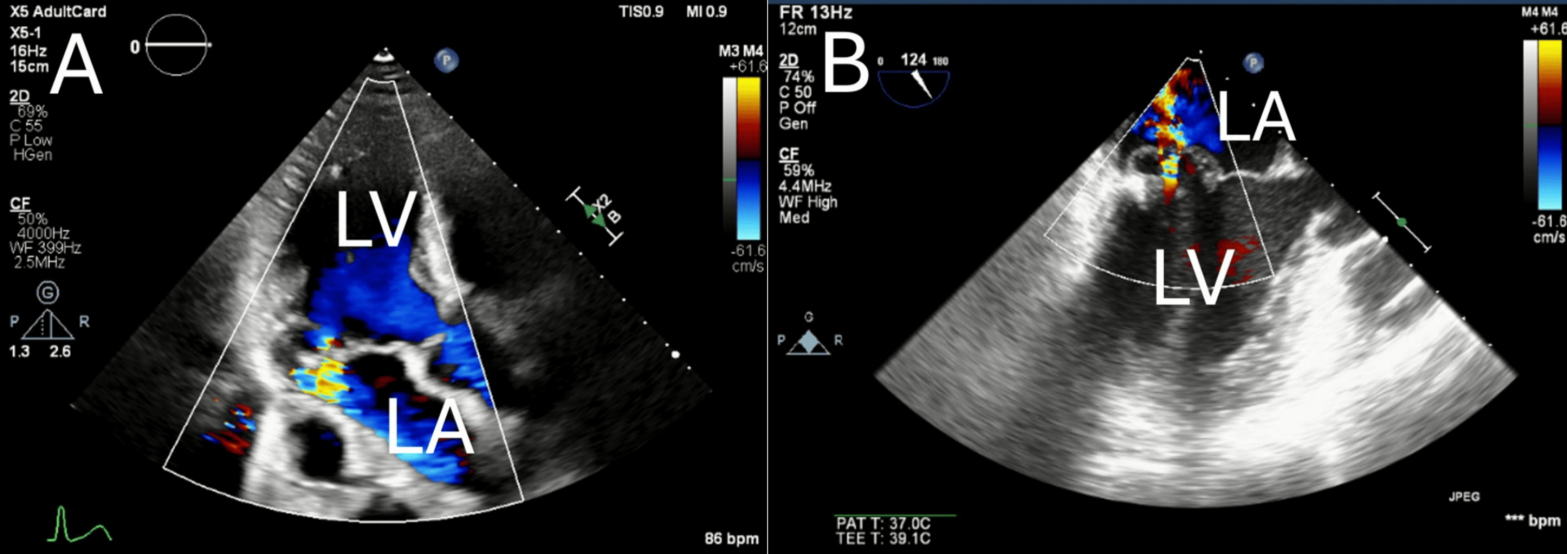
Preoperative echocardiographic findings. (A) Transthoracic echocardiography (TTE) before surgery shows an eccentric jet suggesting anterior leaflet prolapse. (B) Intraoperative transesophageal echocardiography (TEE) before repair reveals prolapse-like motion of both leaflets, consistent with Barlow’s disease.
